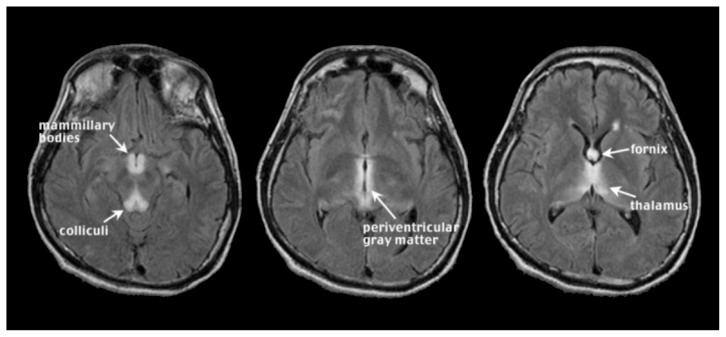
Wernick’s encephalopathy (WE). In acute WE, magnetic resonance imaging (MRI) can detect symmetrical, bilateral hyperintense foci, visible on T2-weighted and fluid attenuation inversion recovery (FLAIR) images, in periaqueductal gray matter, mammillary bodies, and tissue surrounding the third ventricle.
